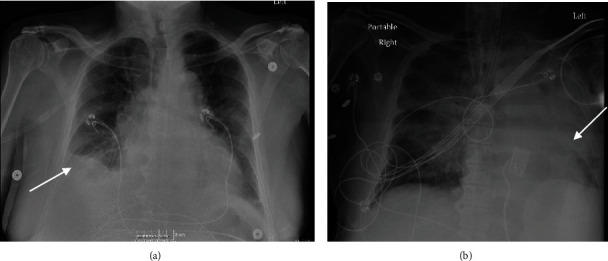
(a) Chest radiograph showing small right pleural effusion on admission. (b) Chest radiograph post-CPR showing large left pleural effusion.
